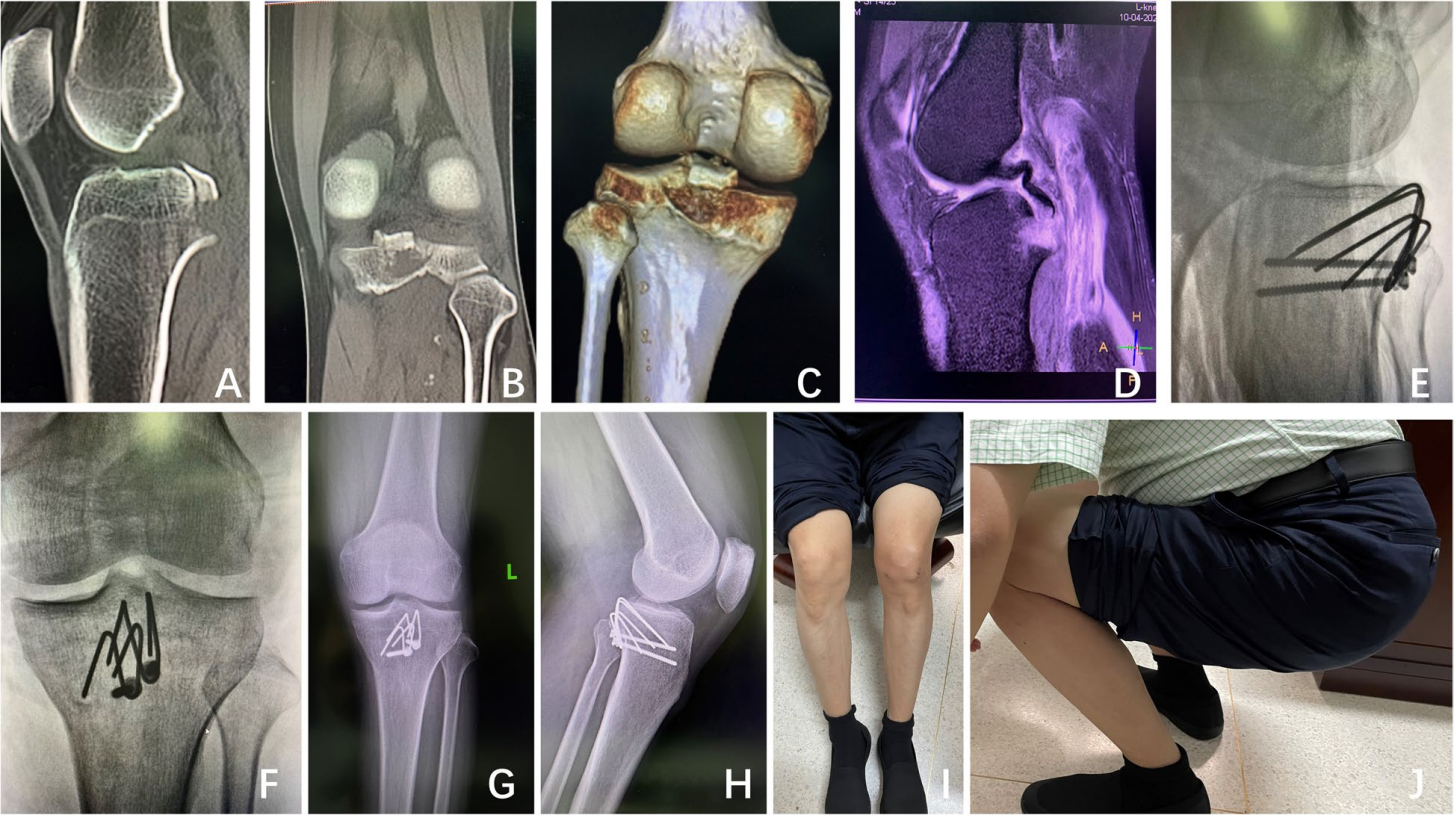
The case I was diagnosed with an avulsed left PCL tibial insertion caused by traffic accidents. A-D Preoperative CT scan and MRI confirming the PCL avulsion fracture; E-F Postoperative fluoroscopy showing the optimum position of the homemade pin-hook and fracture reduction; G-H Three-month postoperative follow-up radiographs showing fracture union and no loosening of internal fixation. I-J Three-month postoperative photos displaying good knee function without any discomfort
